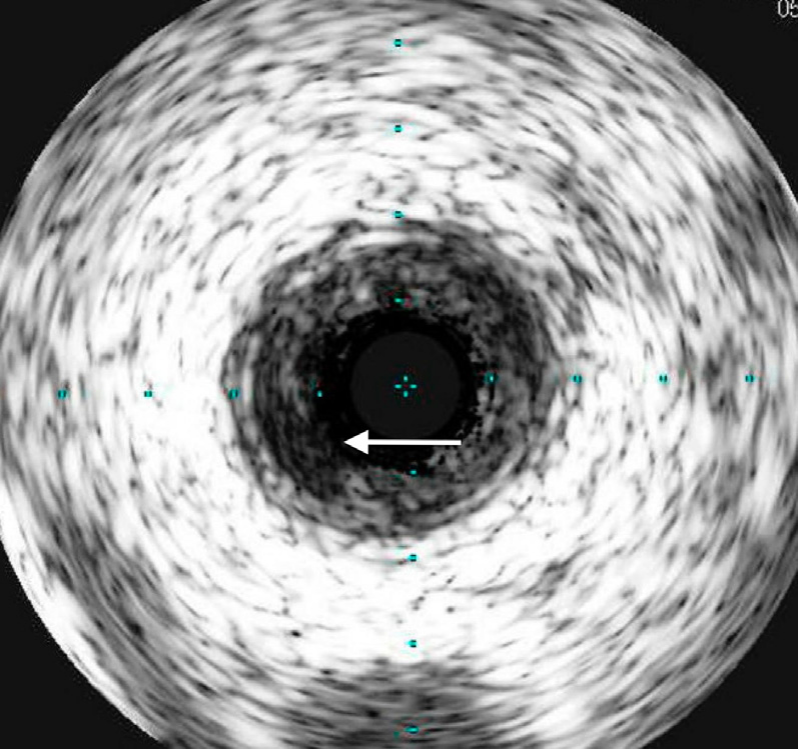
Intravascular Ultrasound Intravascular ultrasound demonstrated a left anterior descending coronary artery free of any atherosclerosis except a very short segment, approximately 10 mm long, in the proximal segment with ruptured plaque (white arrow).
